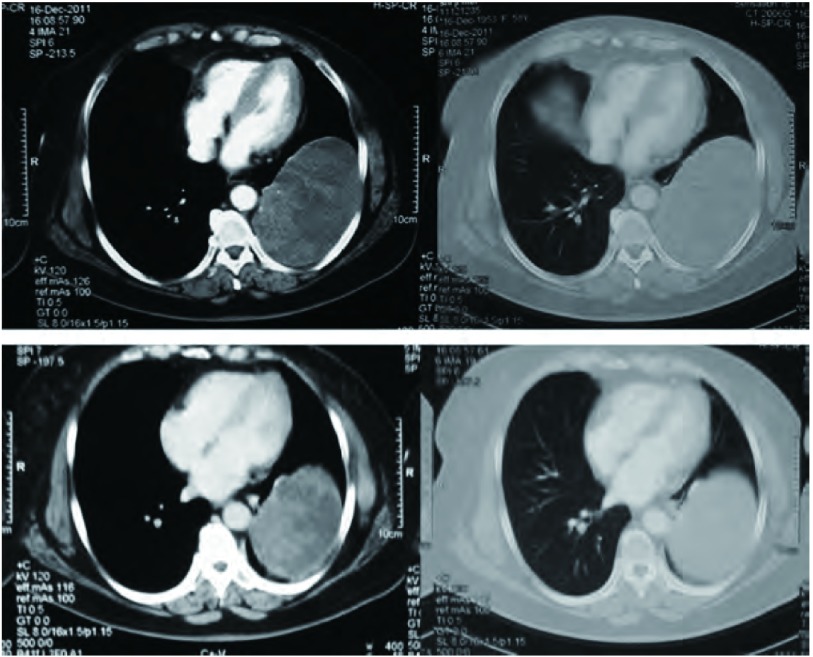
胸部CT增强示左下肺有一大小约13 cm×10 cm×8 cm的占位，边界光整，病灶中实性部分呈轻度强化征象。 Contrast enhanced CT scan of the chest: a large mass (13 cm×10 cm×8 cm) in the left lower lobe, with clear boundaries and smooth fringes. The solid portion of the mass shows a slight contrast enhancement.
